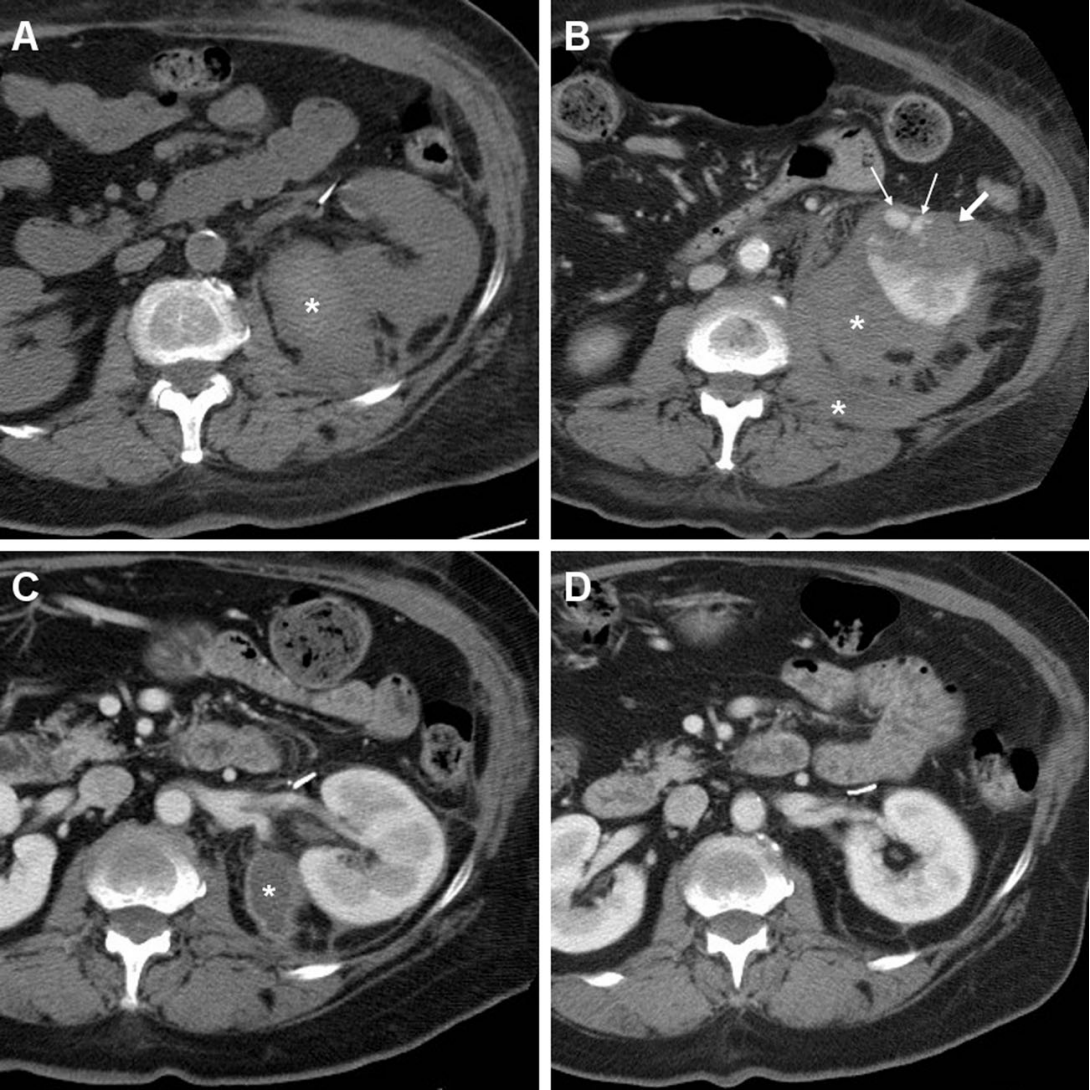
Abdominal pain and hypotension in a 62-year–old woman 24 h after laparoscopic enucleation of an early-stage RCC. Unenhanced CT acquisition (a) showed renal displacement by hyperattenuating medial peri-renal and posterior para-renal haematoma (*). Post-contrast corticomedullary-phase images (b) depicted foci of active extravasation (*thin arrows*) near the site of resection (*arrow*) at the resected inferior renal pole. The haematoma (*) become progressively liquefied and demarcated during CT follow-up (c) and eventually resolved 1 year later (d). (Partially reprinted with permission from Tonolini et al. [52])
